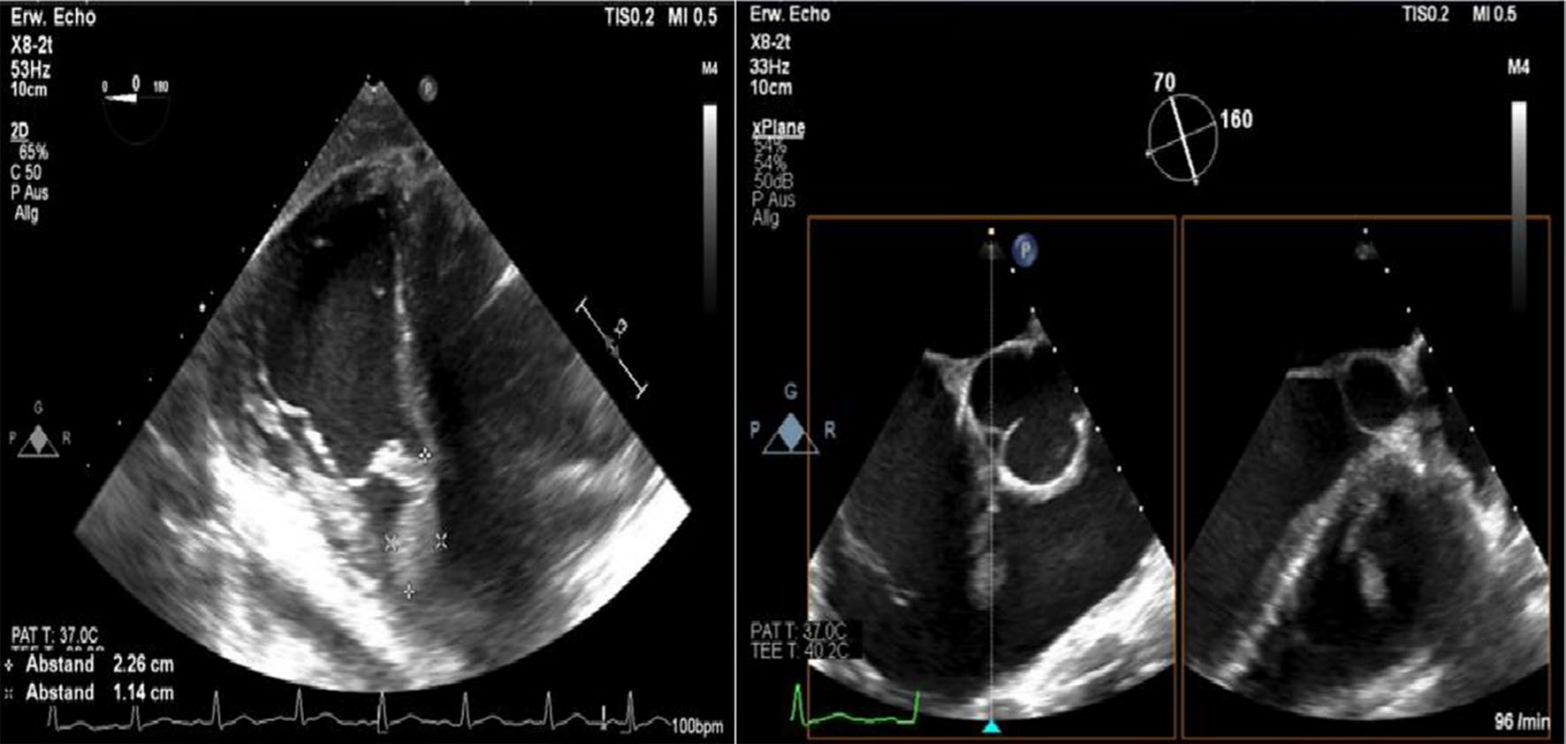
Initial transoesophageal echocardiography showing vegetation on the septal leaflet of the tricuspid valve
